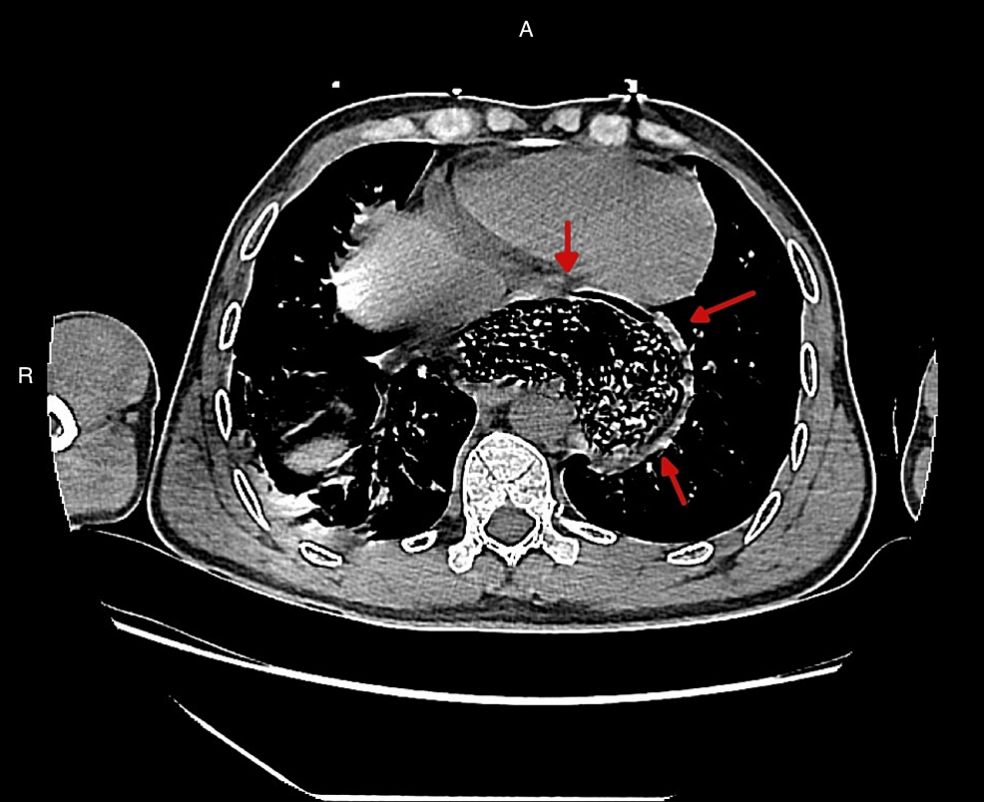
Transaxial chest CT without contrast. There is a severely dilated esophagus (red arrows) and a significant amount of air and residual food debris can be seen.
